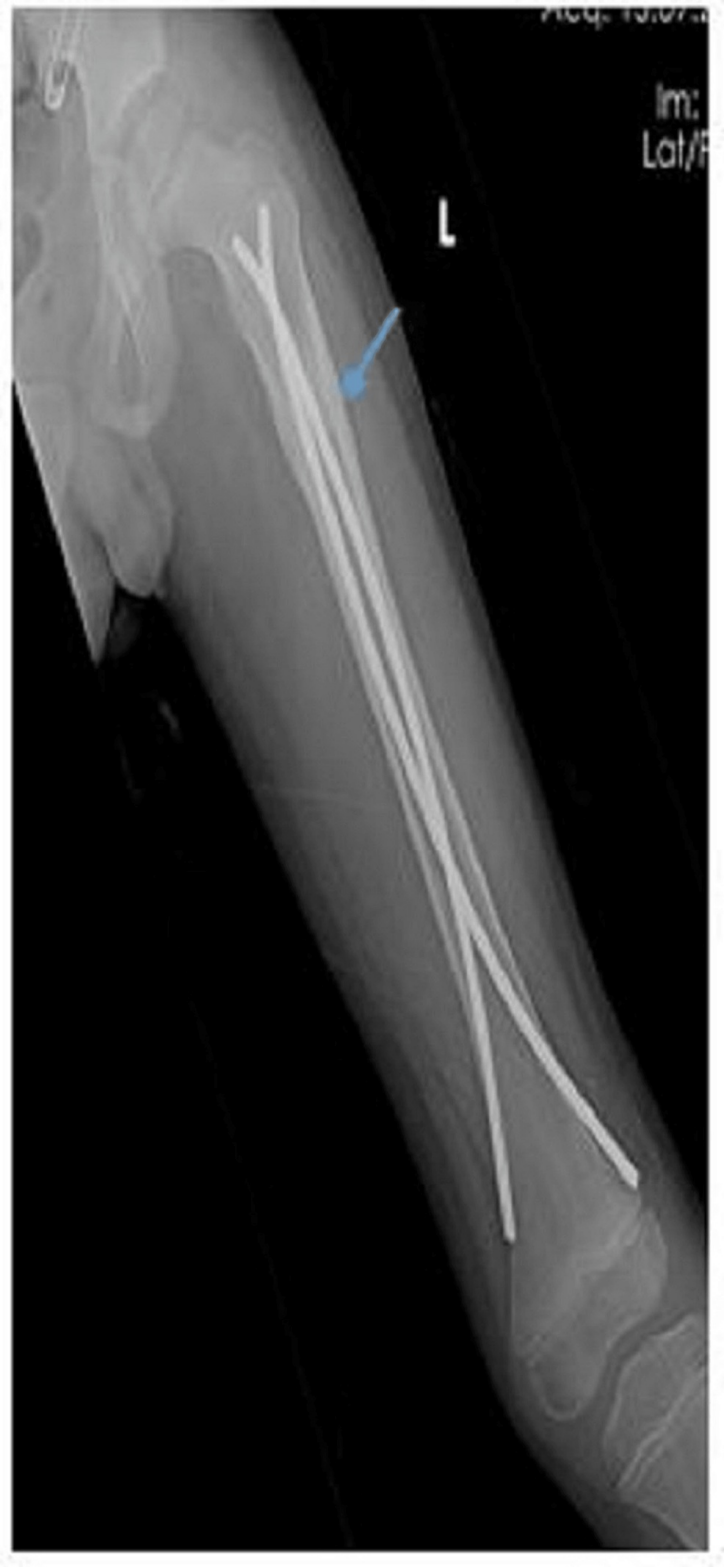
Antero-posterior radiograph of a nine-year-old male who underwent TENS fixation for subtrochanteric fracture (blue arrow) with fracture union TENS: titanium elastic nailing system
